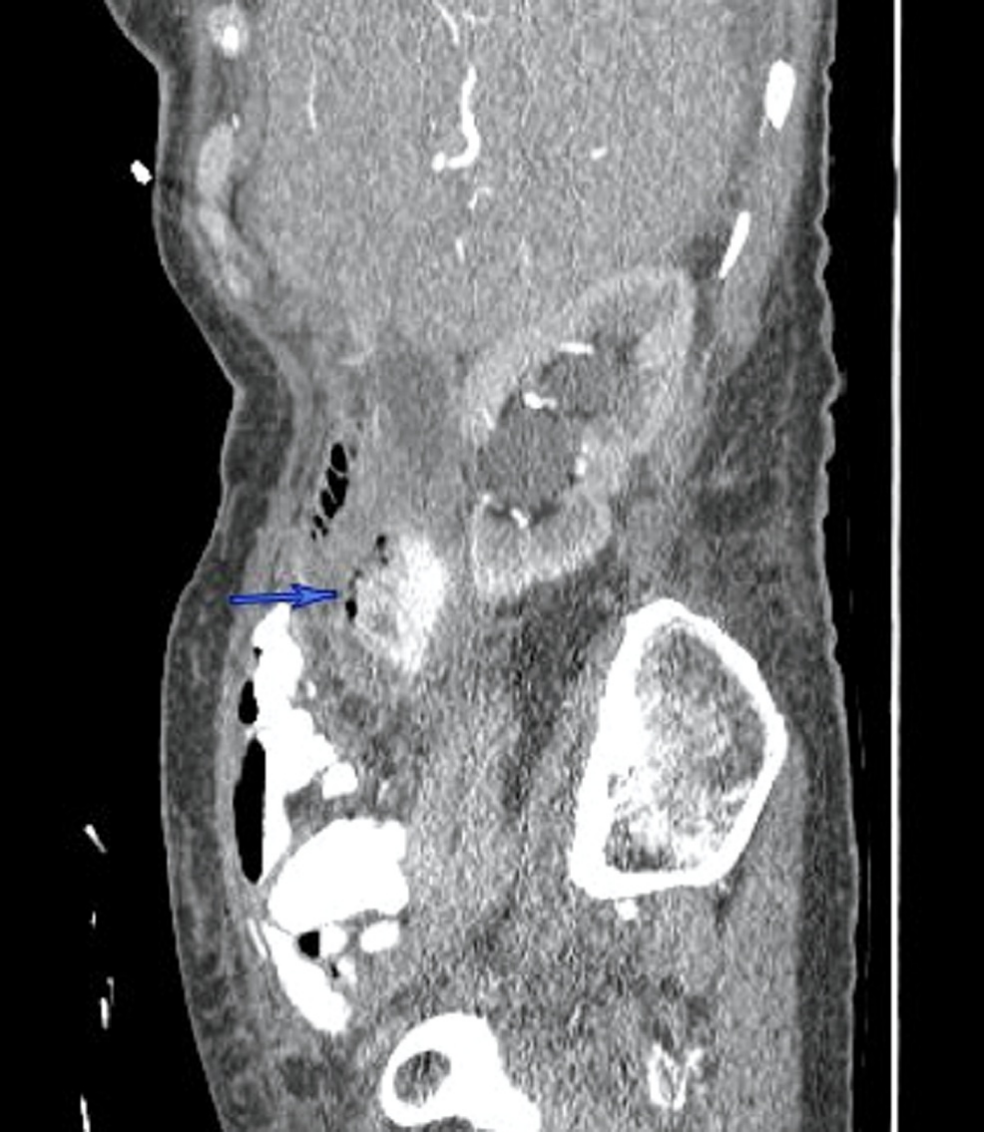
CT abdomen (sagittal section) demonstrates a complex collection with a contained contrast leak related to the perforated duodenal diverticulum
